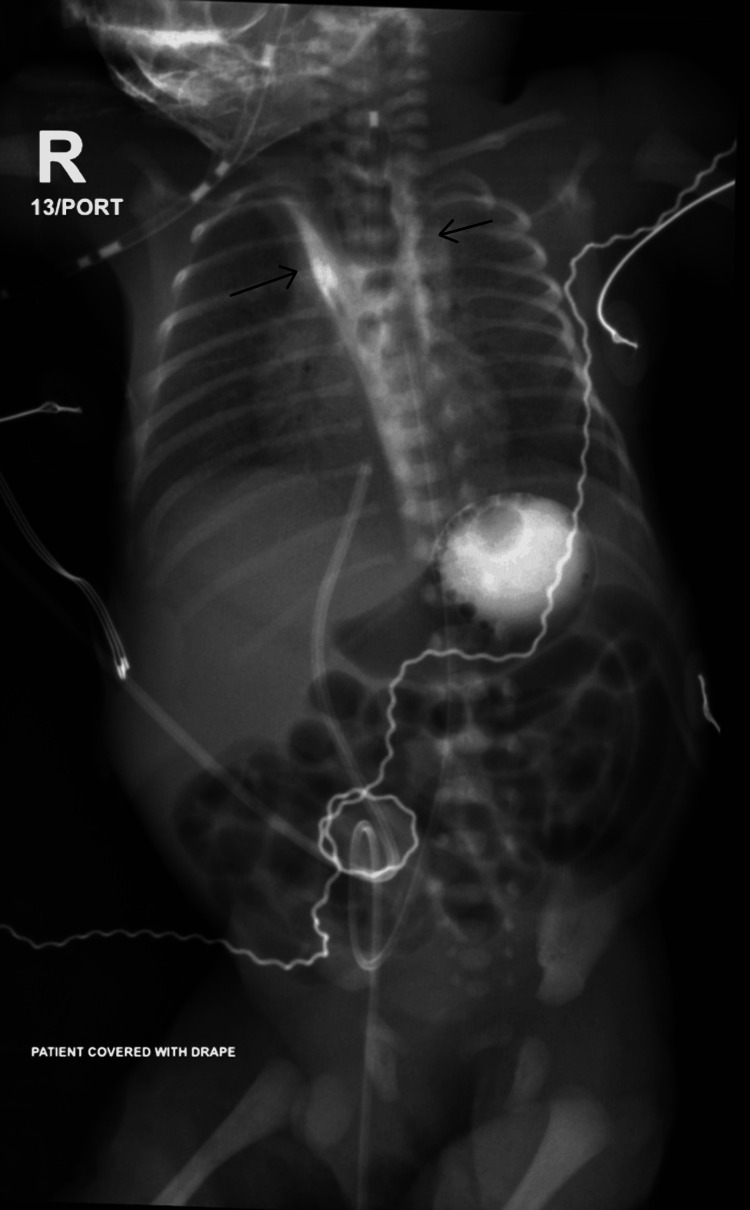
Contrast-enhanced X-ray showing contrast leak into the mediastinum suggestive of EP Contrast-enhanced X-ray shows contrast material within the mediastinum with contrast extravasation into the mediastinal space causing wide mediastinum (the two arrows). EP: Esophageal perforation
